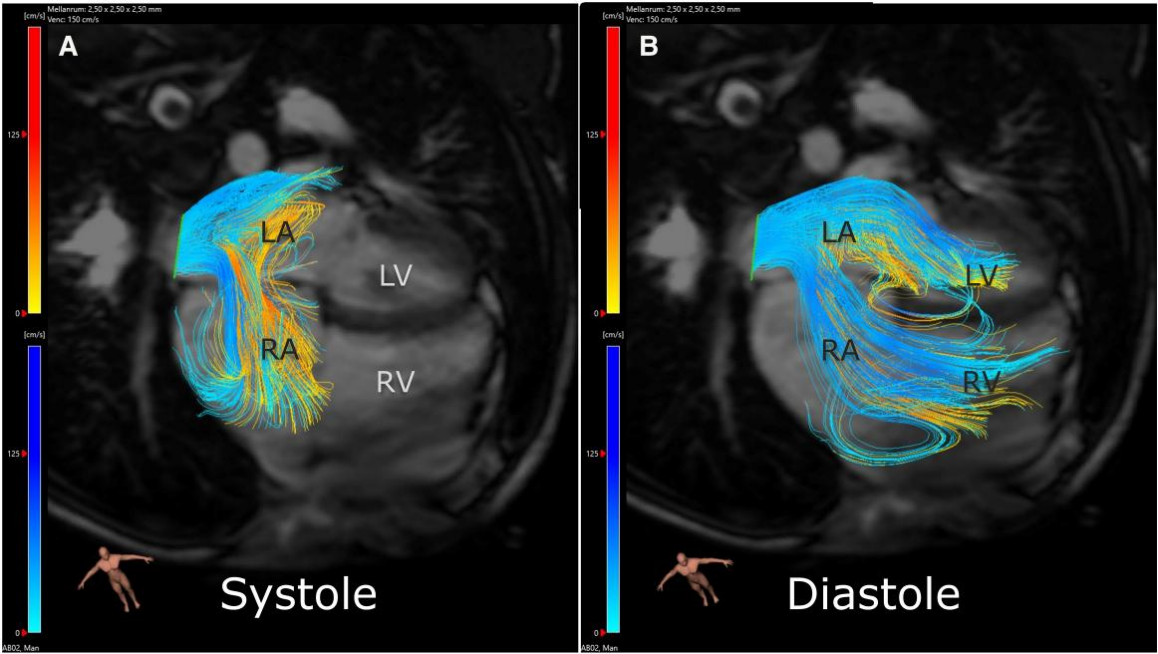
Blood flow through an ASD in a child. Streamlines from four-dimensional flow overlaid on cine images by cardiac magnetic resonance imaging. The yellow streamlines show the flow from the left pulmonary veins, and the blue streamlines show the flow from the right pulmonary veins. The left-to-right shunt is visualized in systole (*A*) and in diastole (*B*). LA, left atrium; LV, left ventricle; RA, right atrium; RV, right ventricle.
